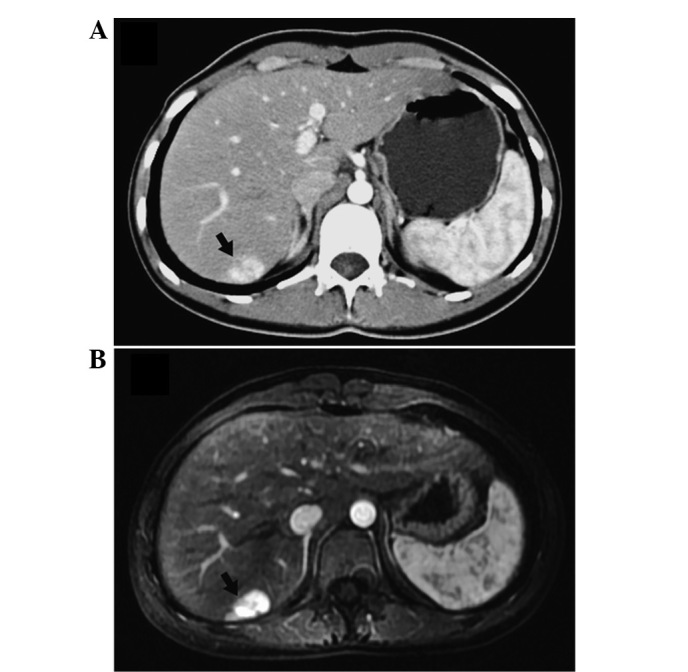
Imaging of hepatic PEComa. (A) Contrast-enhanced phase of CT reveals a poorly-defined mass with a significantly high intensity in segment VII of the liver. (B) The liver mass in segment VII revealed a strong enhancement in the contrast-enhanced phase of the MRI. PEComa, perivascular epithelioid cell tumor; CT, computed tomography; MRI, magnetic resonance imaging.
